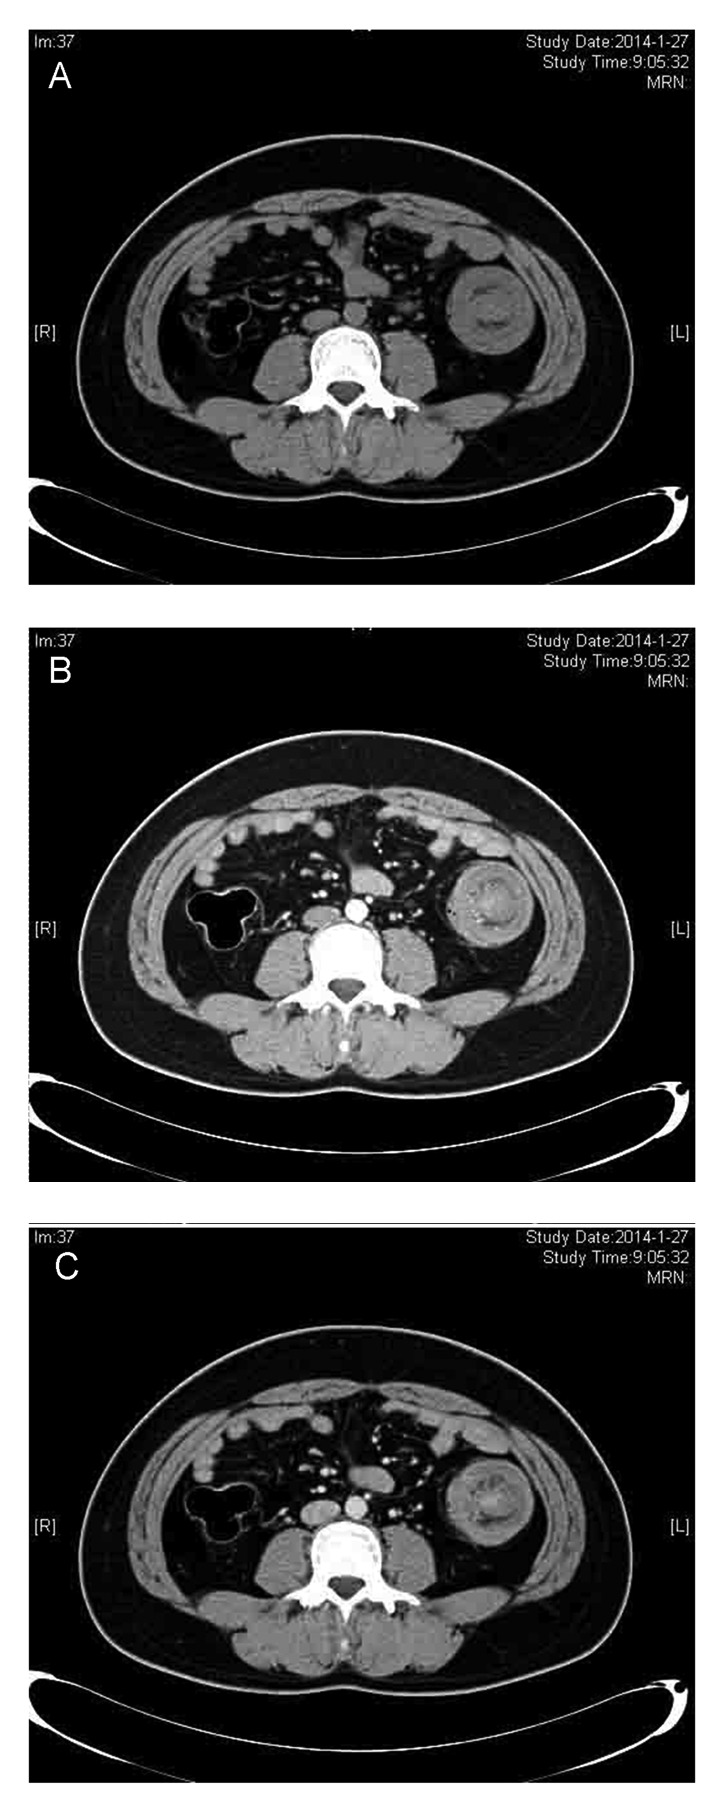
A) Unenhanced, (B) arterial phase and (C) portal venous phase computed tomography images revealing a typical target lesion associated with descending colo-colonic intussusception.
